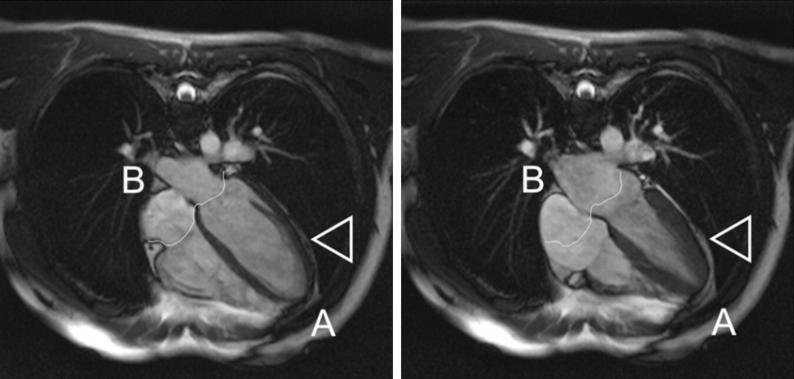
MRI of a human heart in end-diastole (left) and end-systole (right). Note stable position of apex and base (A, B), and minimal lateral translocation of epicardial boundaries (arrow-head). Intra-ventricular volume reduction is dominated by (i) pronounced centripetal tissue thickening, and (ii) the baso-apical shift of the atrio-ventricular valve-plane (see white outline). This is different from the pronounced apex “skipping” seen in isolated coronary-perfused hearts, where the removal of pericardial constraints (which provide a pleura-like viscous coupling that allows for ventricular twisting and sliding, but prevents tissue disconnection) changes cardiac pump function from the normal mode of combined ventricular pressure and atrial suction generation to a ventricular pressure only mode. Modified from (Petersen, 2006); with permission.
